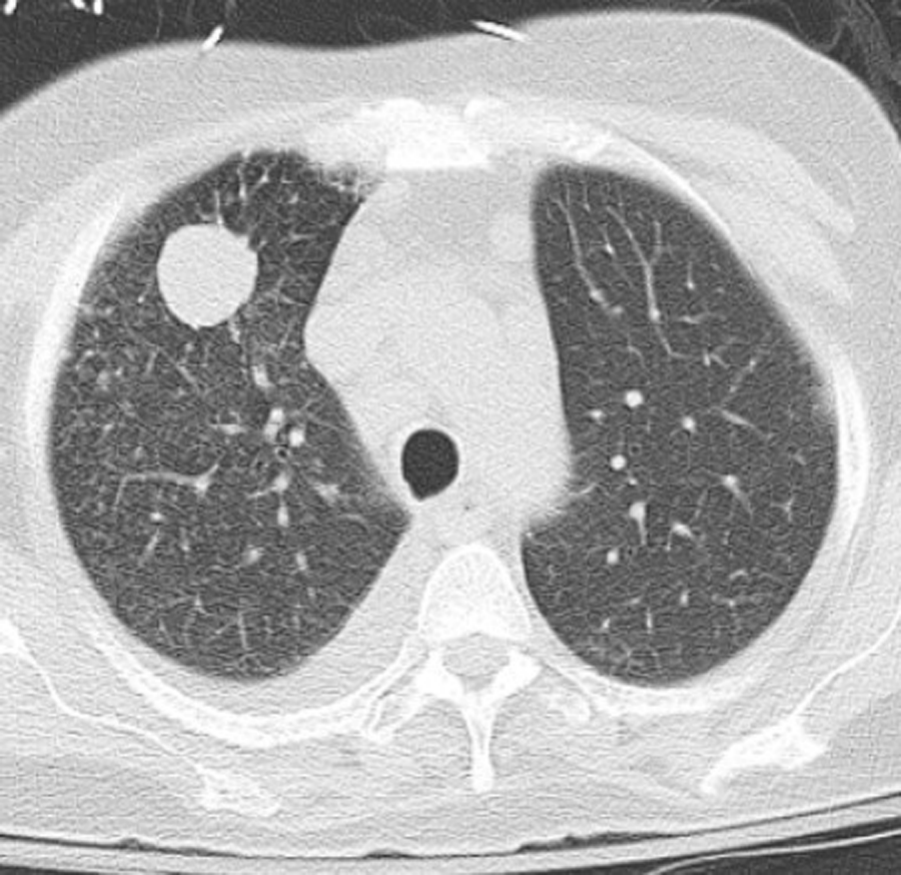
CT chest without contrast demonstrated a solitary cavitary pulmonary lesion in the right lung, associated with a small pleural effusion.
